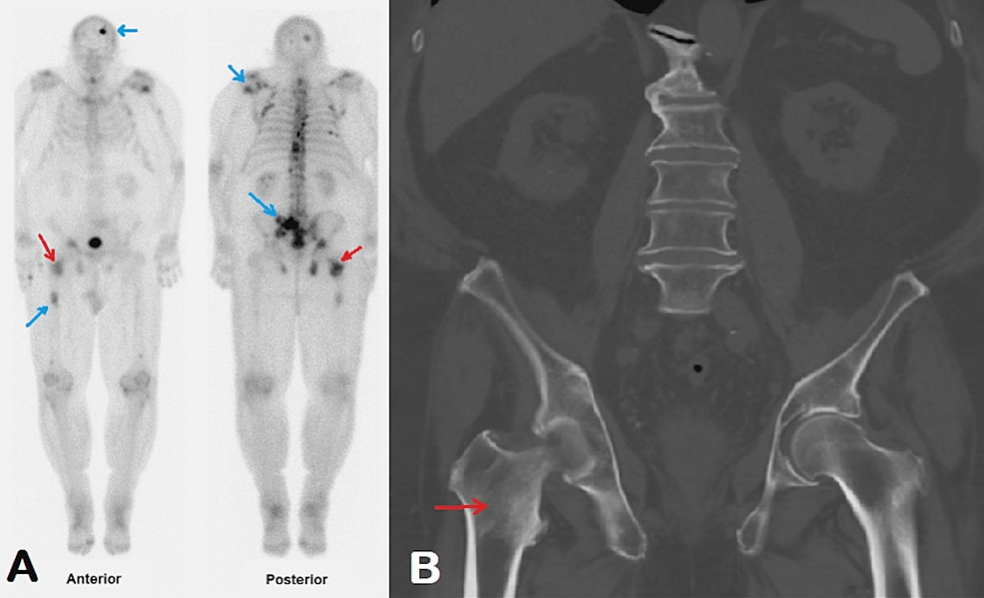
Findings on baseline imaging in October 2020 (A) Technetium-99m MDP whole-body bone scan shows multifocal abnormal radiotracer uptake in the skeleton (blue arrows), compatible with diffuse osseous metastatic disease; (B) CT without intravenous contrast shows multiple sclerotic lesions in the skeleton, compatible with bone metastases. The sclerotic lesion in the right intertrochanteric region on CT shows corresponding bone scan uptake (red arrows). MDP - methylene diphosphonate
